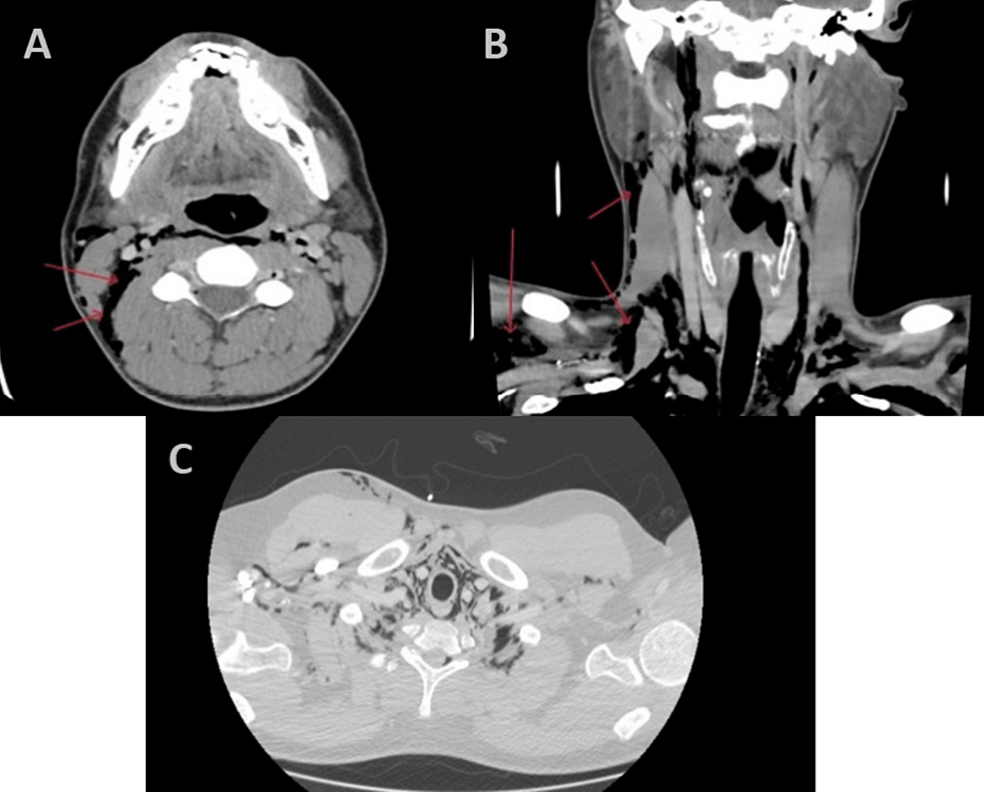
CT showing air collection within the soft tissue of the neck.
